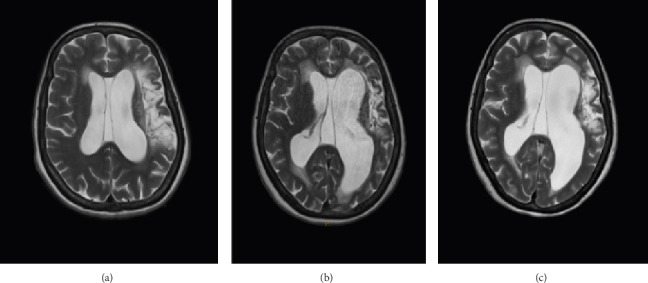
(a) As a comparison, an MRI of the brain 3 years prior to admission showed an established large left MCA territory infarct and hydrocephalus ex vacuo phenomena of the left lateral ventricle. (b) MRI of the brain performed approximately 1.5 months prior to admission showed significant encephalomalacia and gliosis involving the left frontal lobe, resulting in ex vacuo dilatation of the left lateral ventricle and asymmetric ventriculomegaly. (c) A follow-up MRI of the brain, conducted 2 weeks after admission—approximately 2 months after the previous scan (Figure 1(b))—demonstrated persistent chronic compensated hydrocephalus with an extensive left MCA territory infarct, without evidence of meningoencephalitis or cryptococcomas.
